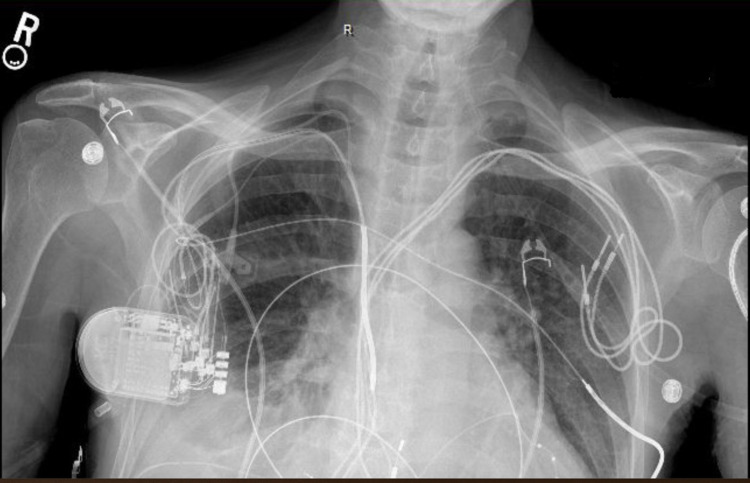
Chest X-ray showing right pleural effusion with adjacent atelectasis.
